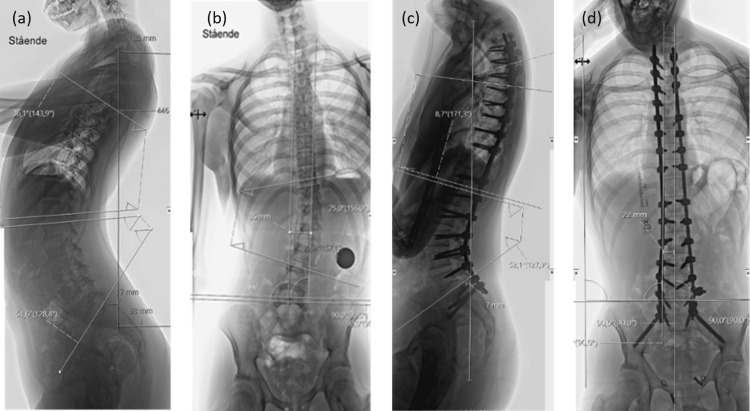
Preoperative standing radiology lateral (a) and AP (b) spine X-rays showing scoliosis and hyperlordosis. Postoperative standing radiology lateral (c) and AP (d) spine X-rays.
